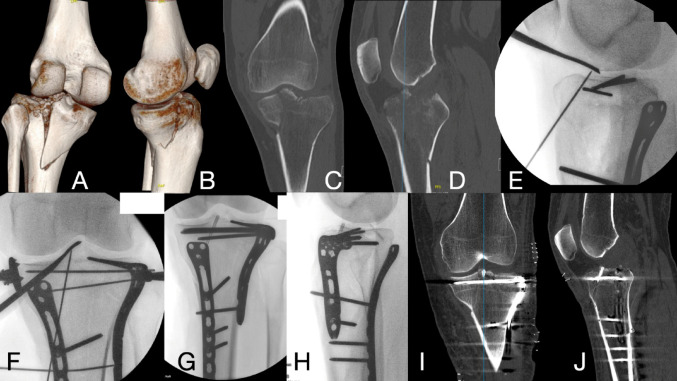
Computed tomography images, including three-dimensional reconstructions (A, B), coronal (C) and sagittal (D) views, revealed a flexion-varus type tibial plateau fracture with a bony avulsion fracture of the ACL. The patient was positioned supine, and surgery began with an anterolateral approach. A lateral tibial condyle osteotomy was performed to expose the depressed joint surface and lateral meniscus. There was no meniscal entrapment or tear, and the posterior root was intact. The lateral joint surface was reconstructed using two 2.7-mm subchondral screws and the osteotomized condyle was reduced back into position. Next, a posteromedial approach was performed, followed by buttress plating to ensure proper alignment. An anteromedial infrapatellar stab incision allowed the introduction of a small raspatory to reposition the ACL avulsion fragment, which was temporarily fixed with a K-wire (E). Interfragmentary compression was achieved after application of a lateral VA-LCP, restoring the condylar width (F). A second K-wire was used to guide placement of a 4 × 48 mm cannulated screw for definitive fixation of the ACL avulsion fracture (G, H). The knee was found to be stable both osseous and ligamentous on intraoperative assessment. Postoperative CT imaging demonstrated adequate fracture reduction and secure fixation of the ACL avulsion fragment (I, J)
